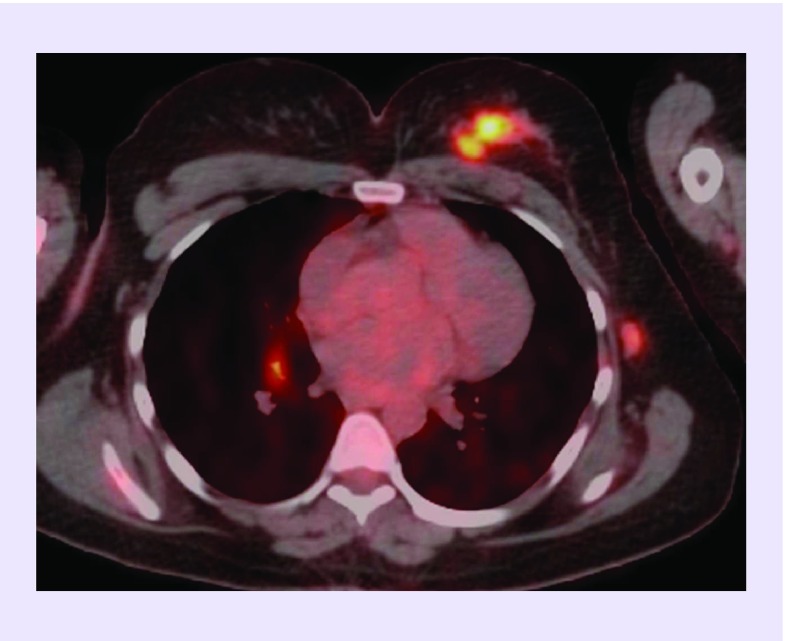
Transaxial fused F-18 fluorodeoxyglucose PET/CT image of a patient with left breast primary tumor in diagnostic PET/CT whole body scan who has additional brain, bone and lymph node metastases.
